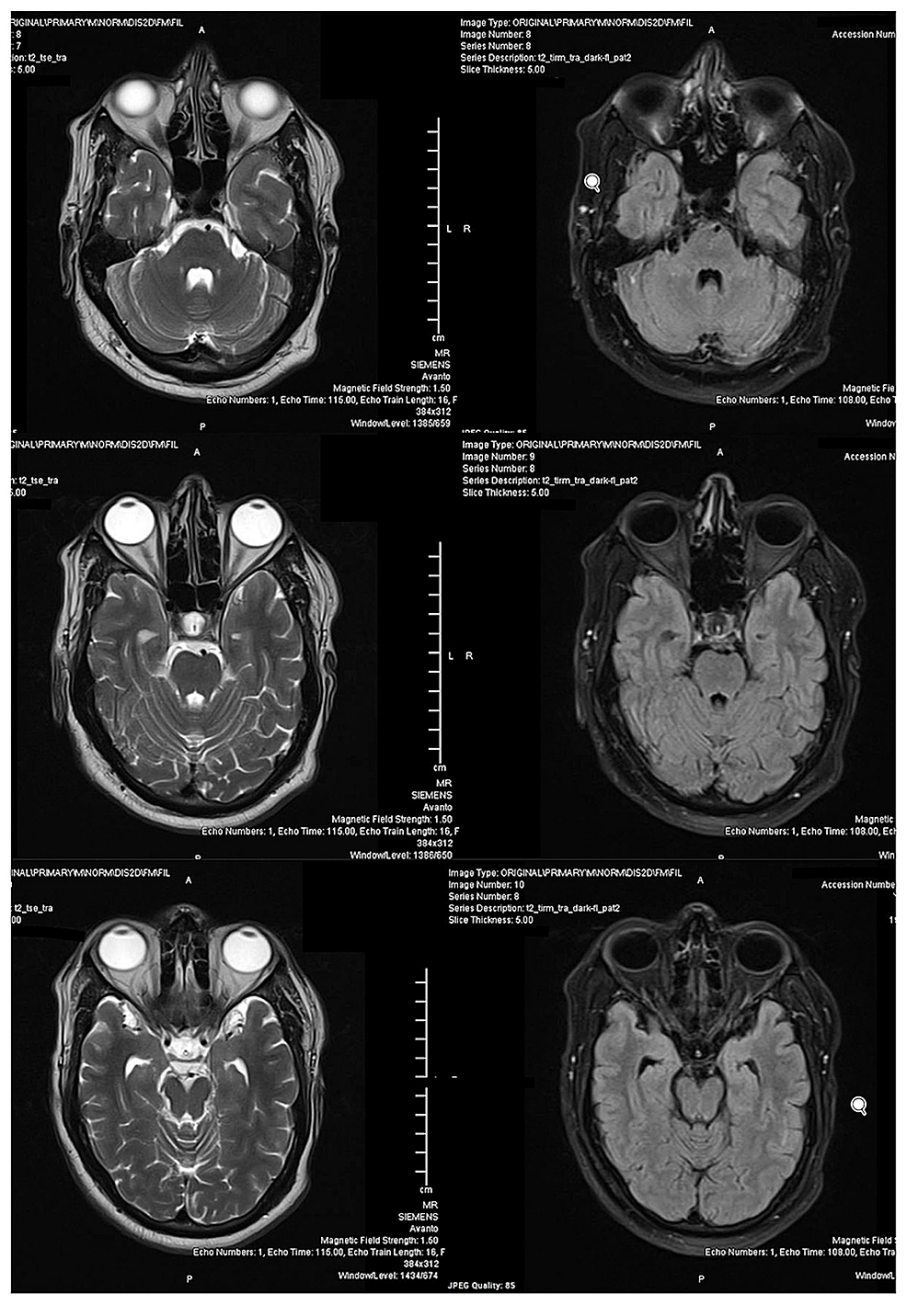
MRI brain of the patient with T2-weighted images (left) and FLAIR images (right) at the level of the pons showing no abnormality. MRI: magnetic resonance imaging; FLAIR: fluid-attenuated inversion recovery
